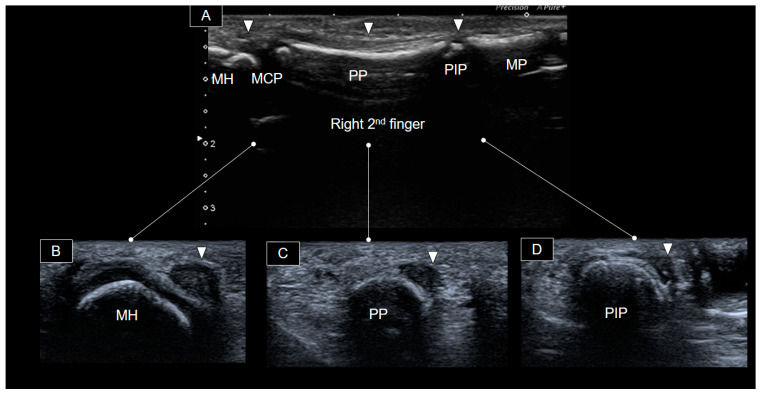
Ultrasound images depicting the palmar aspect of the right 2nd finger in the long axis (A) and at distinct segments (arrowheads) progressing from proximal to distal in the short axis (B–D). MCP, metacarpophalangeal joint; PP, proximal phalanx; PIP, proximal phalangeal joint; MP, middle phalanx; MH, metacarpal head.
